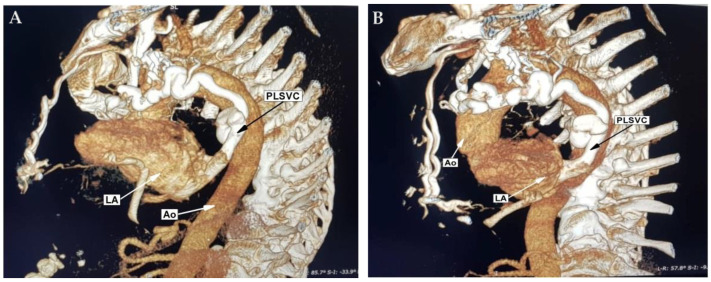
(A,B) Contrast-enhanced computed tomography—3D reconstructed images showing the dilated tortuous PLSVC draining into the left atrium. Abbreviations: LA, left atrium; Ao, aorta; PLSVC, persistent left superior vena cava.
